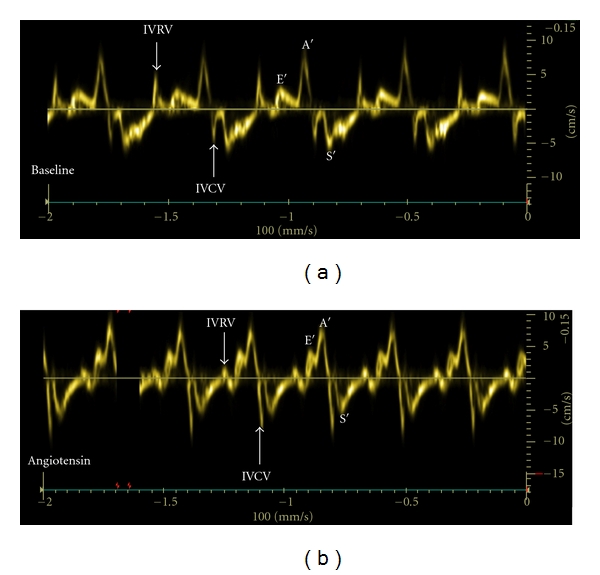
Tissue Doppler-derived left ventricular longitudinal myocardial velocities at the level of mitral valve annulus at baseline (a) and during angiotensin II infusion (b). Note an increase in myocardial isovolumic contraction (IVCV) and early ventricular filling (E′) velocities and a decrease in myocardial isovolumic relaxation velocity (IVRV) during angiotensin II infusion.
